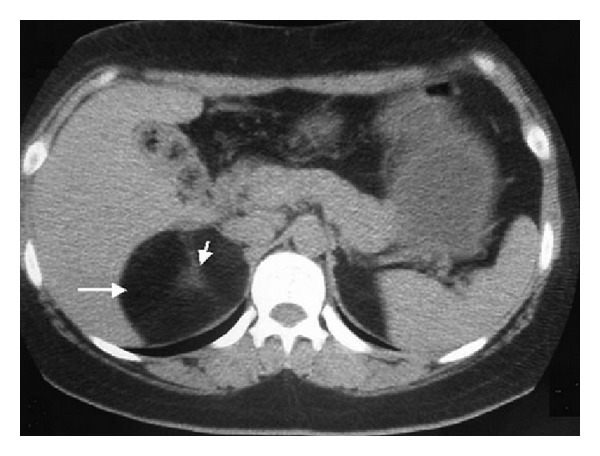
CT appearance of myelolipoma. Contrast-enhanced CT scan of the upper abdomen showing the heterogeneous mass covering upper right retroperitoneal space (arrows) with variable central and peripheral attenuation.
